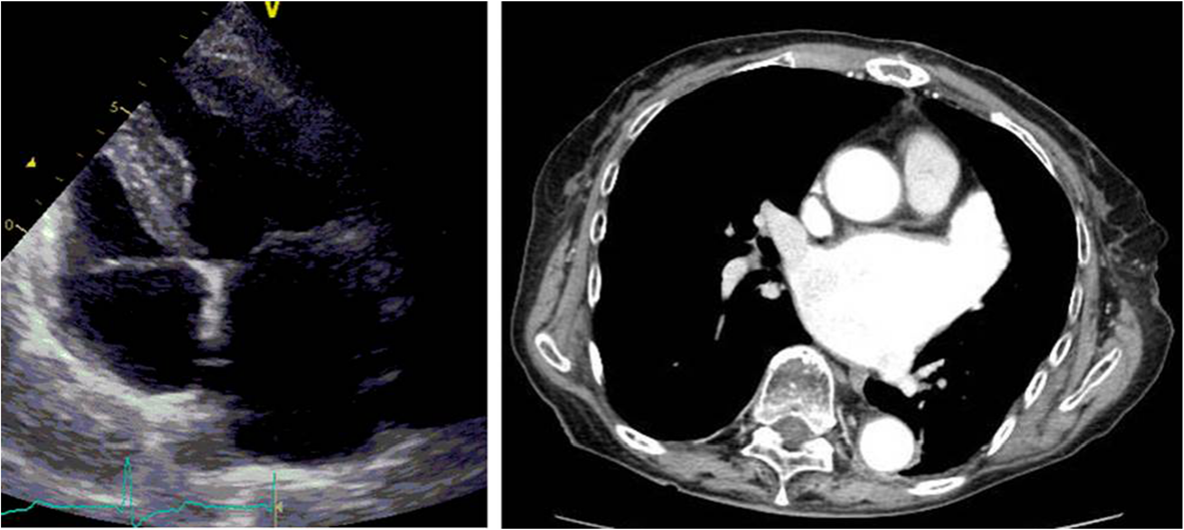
The cardiac thrombus disappeared on transthoracic echocardiography and contrast-enhanced computed tomography of the chest post-apixaban treatment
